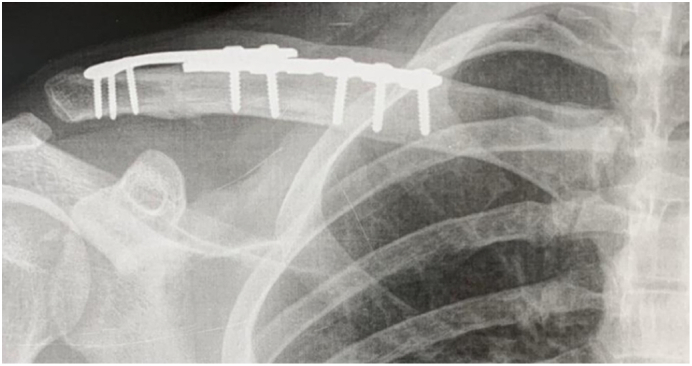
Radiograph at 3-month follow-up showing complete bone healing.
